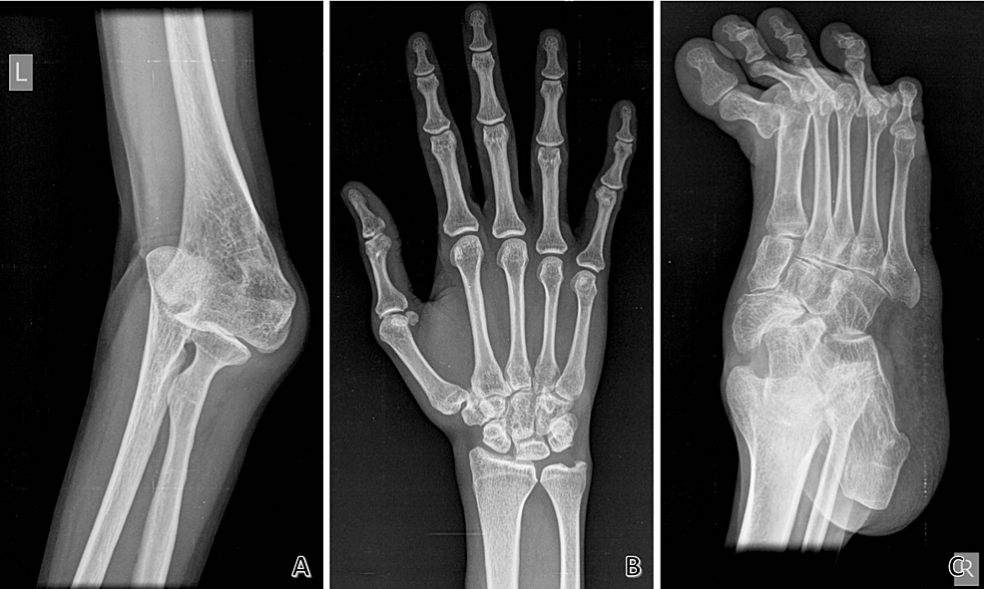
Radiographs The radiographs show subluxation of the left elbow (A), arachnodactyly (B), and dislocations at the right metatarsophalangeal joints (C)
